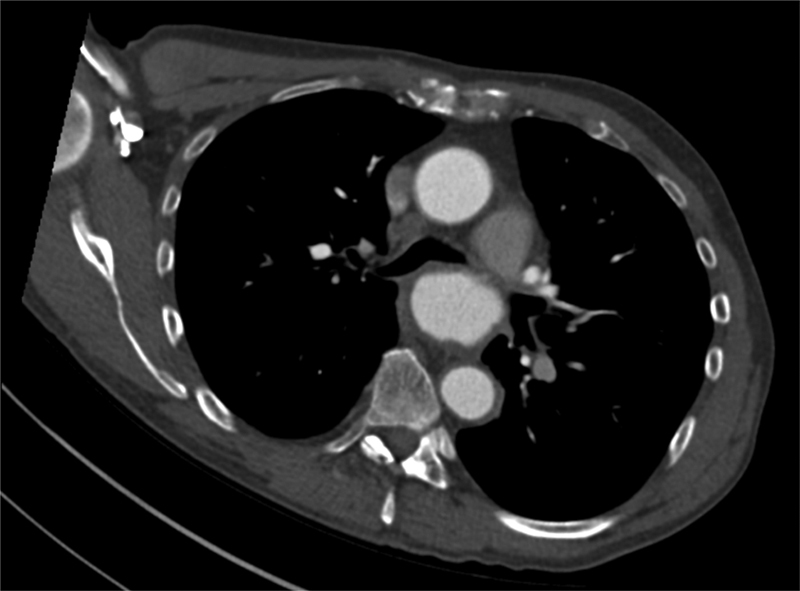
Transverse section of a contrast-enhanced computed tomography scan demonstrating compression of the left main bronchus measuring 2.4 mm at its narrowest.
